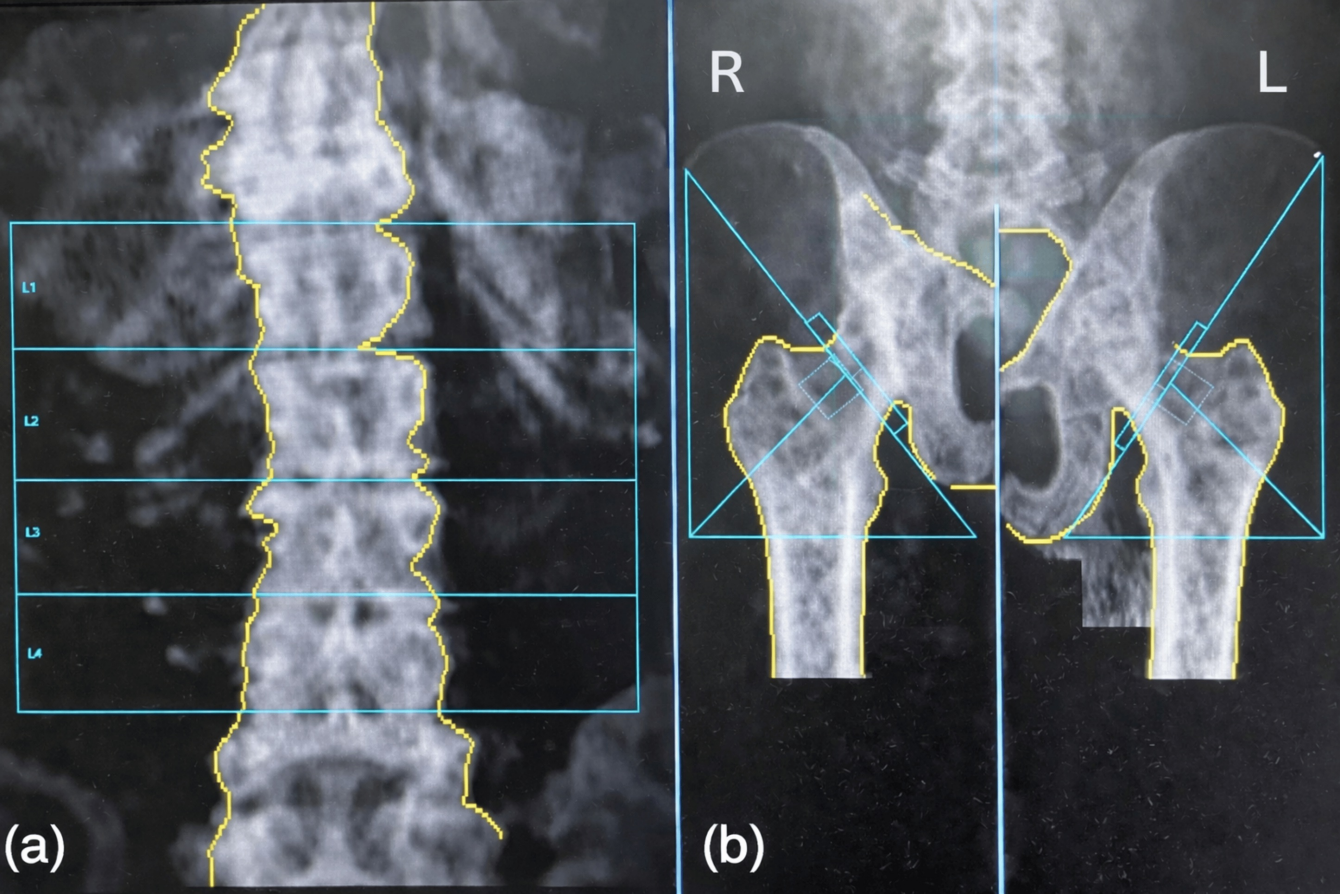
Dual-energy X-ray absorptiometry (DEXA) scan images demonstrating osteoporosis. (a) Anteroposterior lumbar spine (L1–L4) showing reduced bone mineral density with T score of -4.8. (b) Right and left femur showing reduced mineral density over proximal femora with T scores of -2.8 and -3.3, respectively
